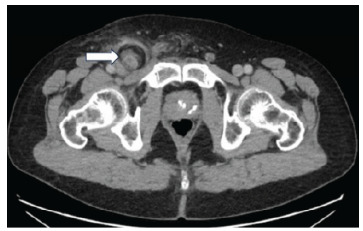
Axial view of computed tomography of the abdomen pelvis showing the appendix within a femoral hernia (arrow).
